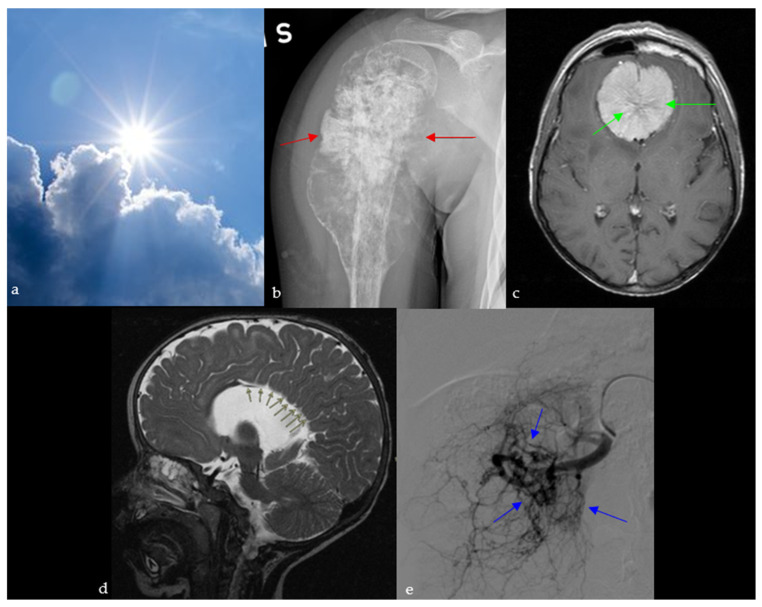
A sunburst appearance observed in nature (a), which resembles the aggressive periosteal reaction with stretching of Sharpey’s fibers perpendicular to the humeral bone on plain radiograph (red arrows) (b), the spoke wheel pattern of vessels in meningioma viewed in cross-section, diverging from dural attachment on brain MRI (green arrows) (c), sagittal T2 images of the brain showing radial orientation of the sulci from the roof of the third ventricle due to dysgenesis of the cingulate gyrus and corpus callosum (green arrows) (d), and the appearance of arterial blush seen at selective arterial DSA of a renal angiomyolipoma (blue arrows) (e).
